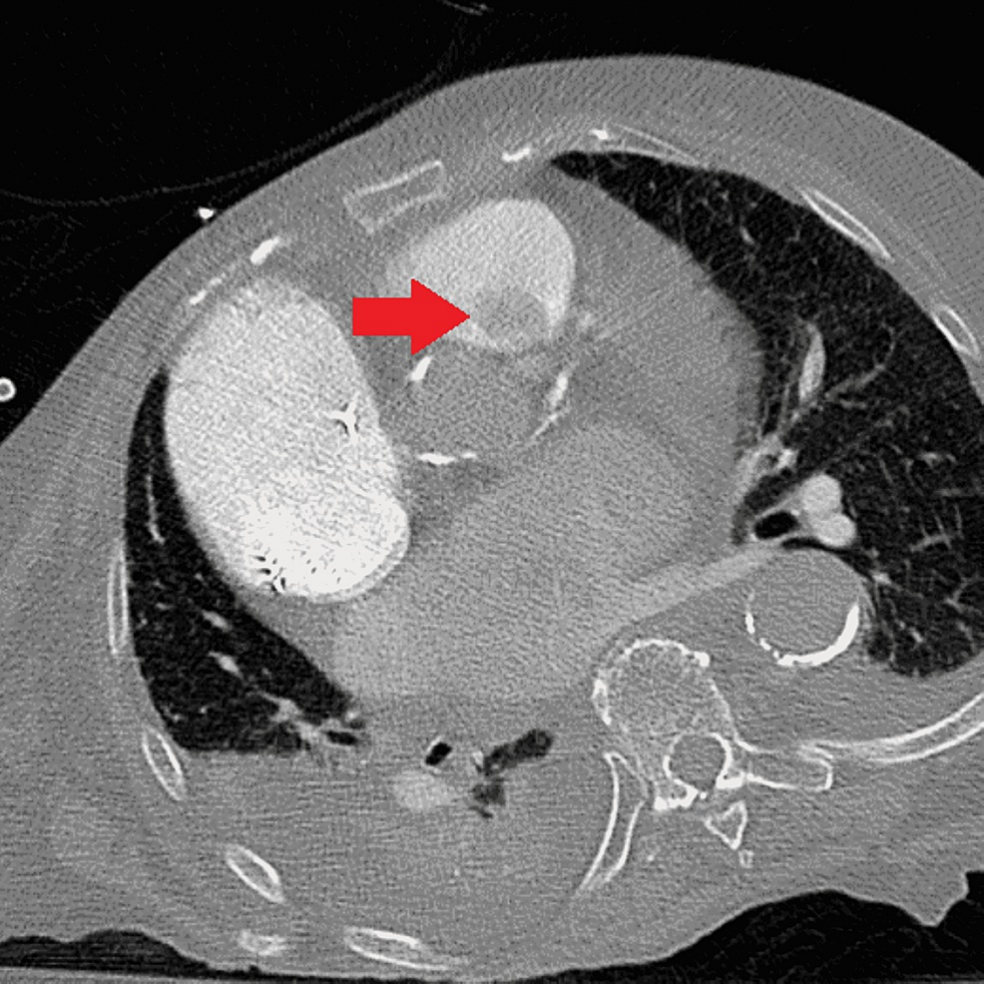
Computed tomography scan showing the papillary fibroelastoma.
